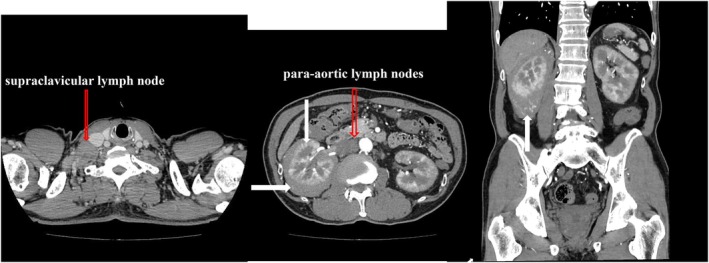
Contrast‐enhanced computed tomography showed that the perinephric tumor was weakly enhanced compared to the renal parenchyma (⇨). The morphology of the right kidney was maintained, and no obvious tumor infiltration into the renal parenchyma was observed (⇩). No necrotic or degenerative findings were observed within the tumor (⇨), and the vascular structure within the tumor was maintained (⇧).
